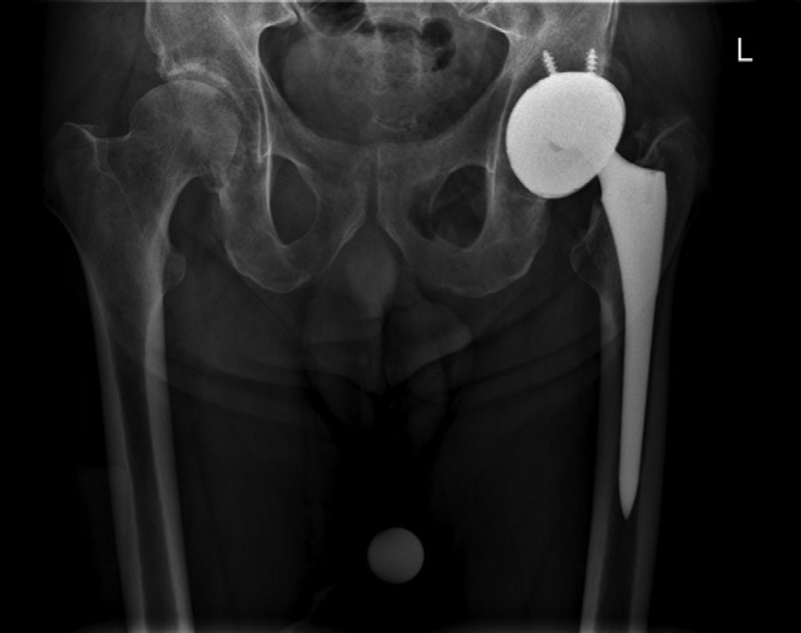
Low-centered AP radiograph of the pelvis shows a left total hip arthroplasty. There is eccentric positioning of the femoral head component within the superior aspect of the acetabular cup.
